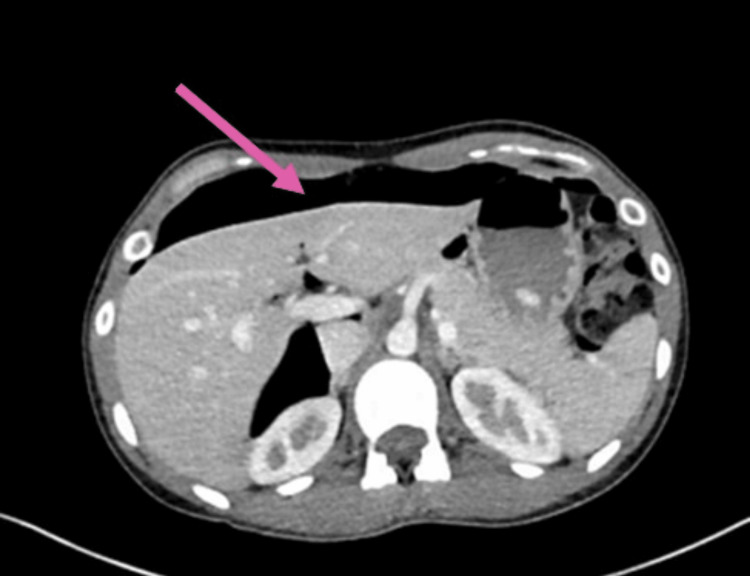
Computed tomography showing pneumoperitoneum, air marked with a pink arrow.
